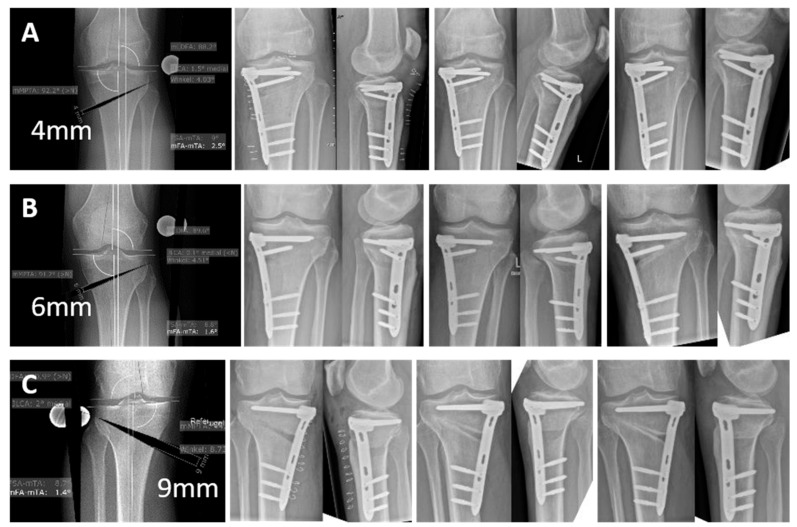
The figure shows 3 exemplary cases of HTOs that were treated with VFLS. The first image in the series shows the pre-operative planning in each case, followed by the X-ray checks post-operatively, after 12 weeks and 6 months. (A) 4 mm opening wedge, (B) 6 mm opening wedge, (C) 9 mm opening wedge.
